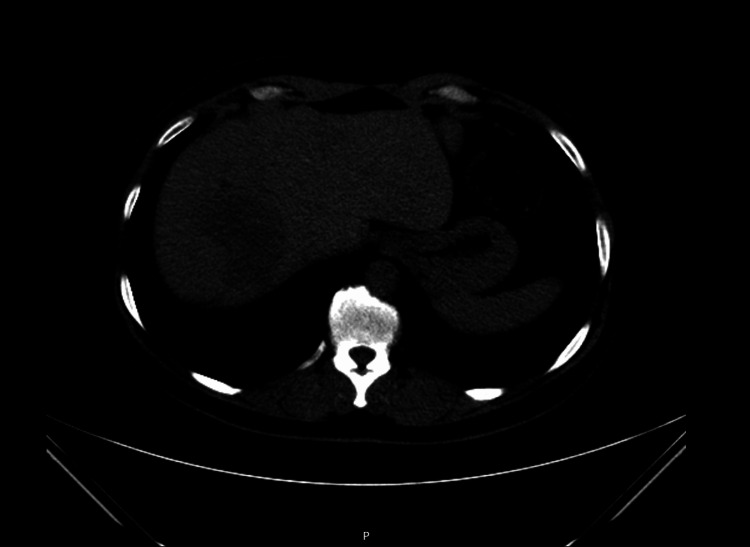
Axial CT image in 2015 showing recurrent hepatic metastases.
